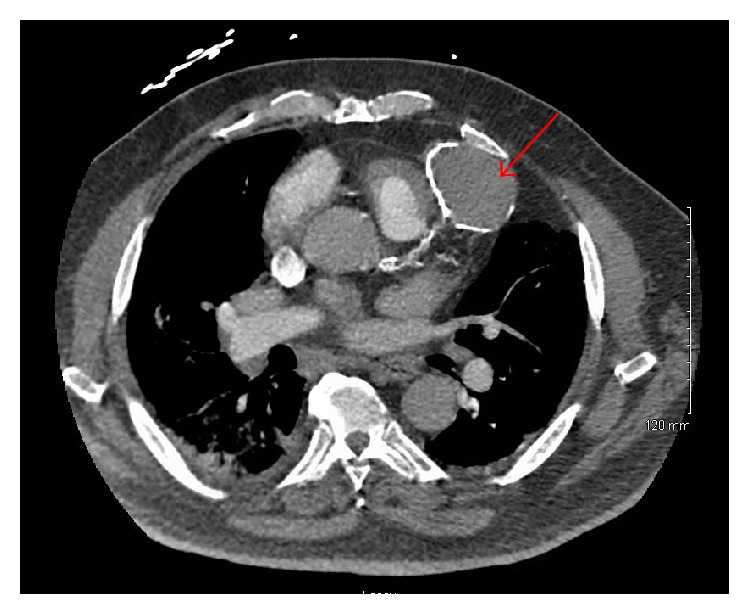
CT pulmonary angiogram.  6.4 × 4.3 cm peripherally calcified hyperattenuating lesion along the intraventricular groove highly concerning for a giant left anterior descending coronary artery aneurysm (red arrow).
